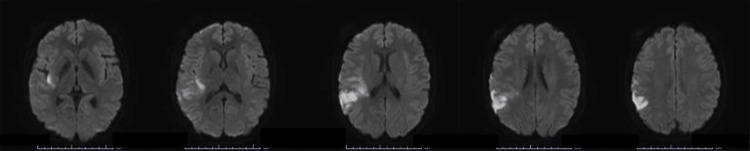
Brain MRI performed on day 0. Axial diffusion magnetic resonance imaging showed high-intensity signals in the right frontal, temporal, and parietal lobes.
